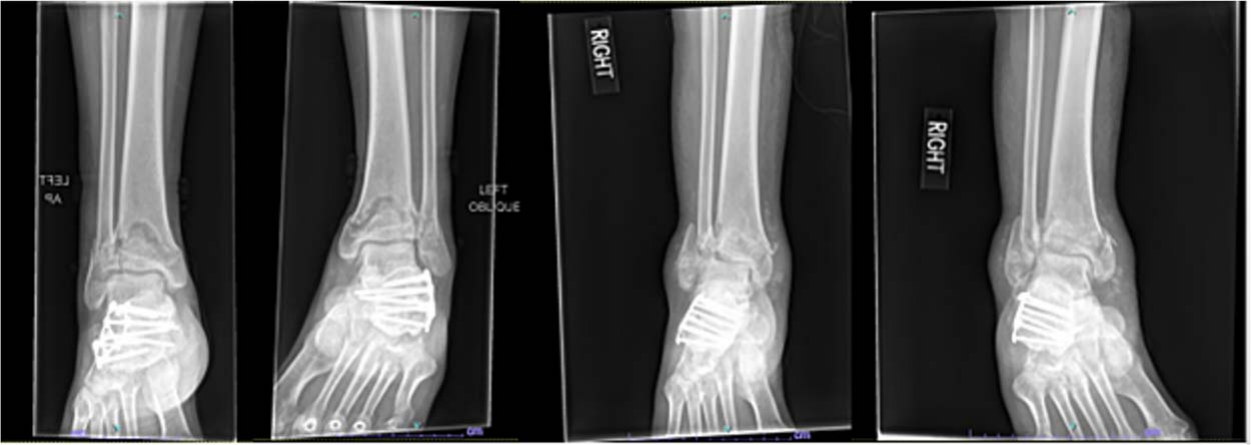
AP and oblique radiographs of patient 2 obtained after a fall, demonstrating bilateral pilon fractures and retained hardware from prior bilateral calcaneal ORIF.
